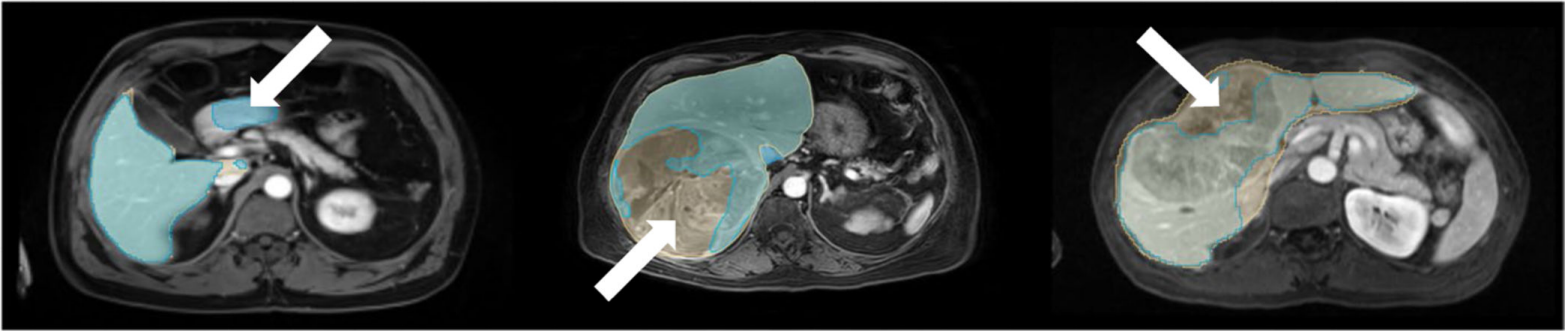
Segmentation failure cases. Liver segmentation failure cases from the internal (left), external (middle), and public (right) test sets with Dice similarity coefficients of 0.945, 0.830, and 0.889 respectively. Axial portal venous phase contrast-enhanced magnetic resonance images are shown with the corresponding manual liver segmentations overlaid in yellow, and the automated segmentations overlaid in blue. In the example on the left, the algorithm segmented parts of the duodenum as liver tissue (arrow). In the example in the middle and on the right, the algorithm excluded parts of a big tumor from the liver segmentation (arrows)
